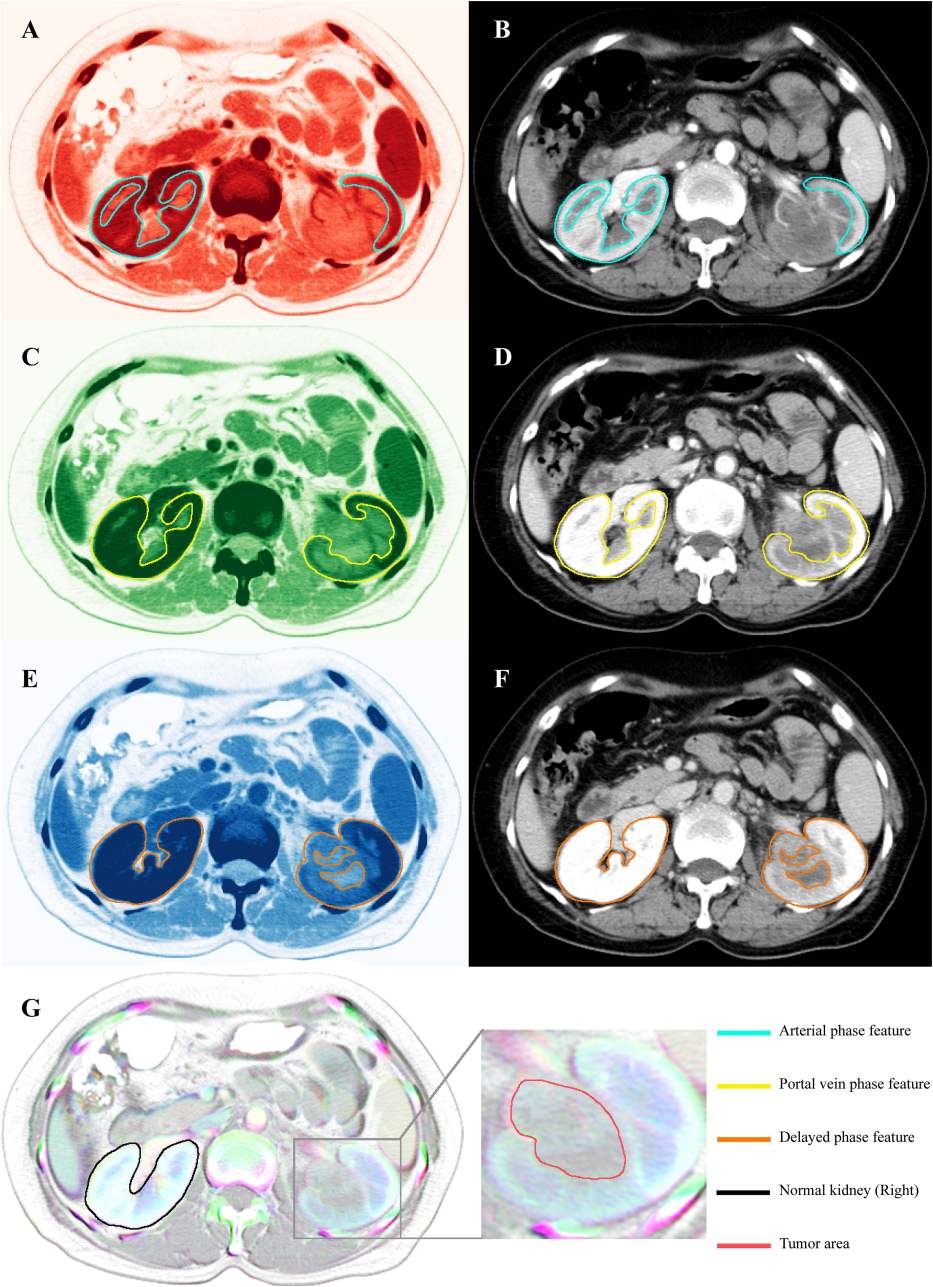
CT scan images and their corresponding features extracted for RGBA color. (B) Original arterial phase CT images and (A) arterial phase images transformed into RGBA (red) through feature extraction. The feature within the green-lined area represents the area of interest in the arterial phase. (D) Original portal vein phase CT images and (C) RGBA (green) images of the portal vein phase. The feature within the yellow-lined area represents the area of interest in the portal vein phase. (F) Original delayed phase CT images and (E) RGBA (blue) images of the delayed phase. The feature within the orange-lined area represents the area of interest in the delayed phase. (G) RGBA image created by the fusion of three phases of CT contrast images (red, green, and blue) and CT plain scan images (alpha). The area marked with a black line represents normal kidneys, distinguished by a white overlay of features from all channels. The enlarged section identifies the tumor region, distinguished by a gray area with central blue-green bands, which effectively captures the radiological changes across the four phases.
